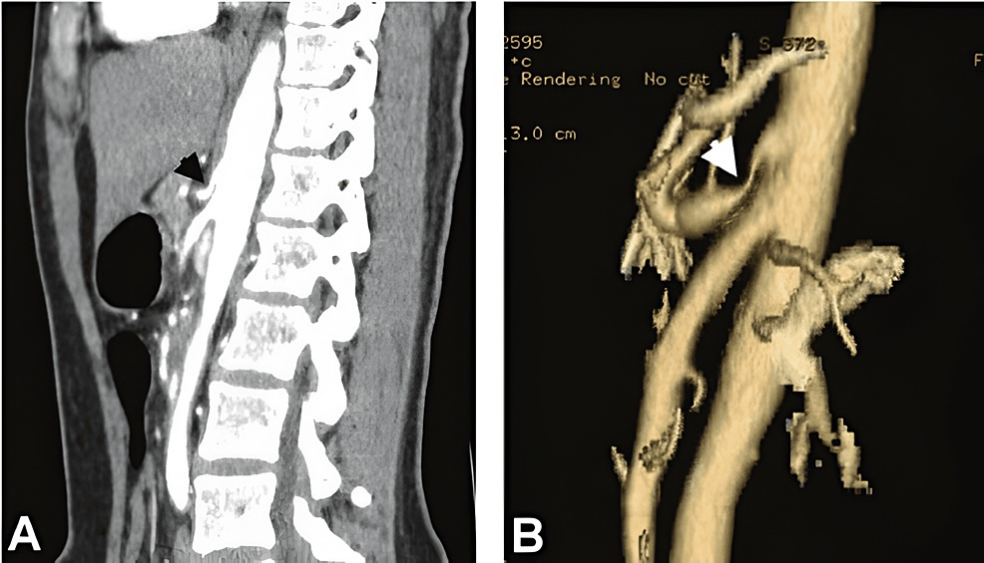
2D and 3D representations of a CTA revealing stenosis of the proximal celiac artery (A black arrow, B white arrow), establishing a diagnosis of MALS. CTA: computed tomography angiogram; MALS: median arcuate ligament syndrome Figure Source: Chou et al., 2012 [8]; reproduced under the Creative Commons Attribution License (CC BY 3.0 Deed)
